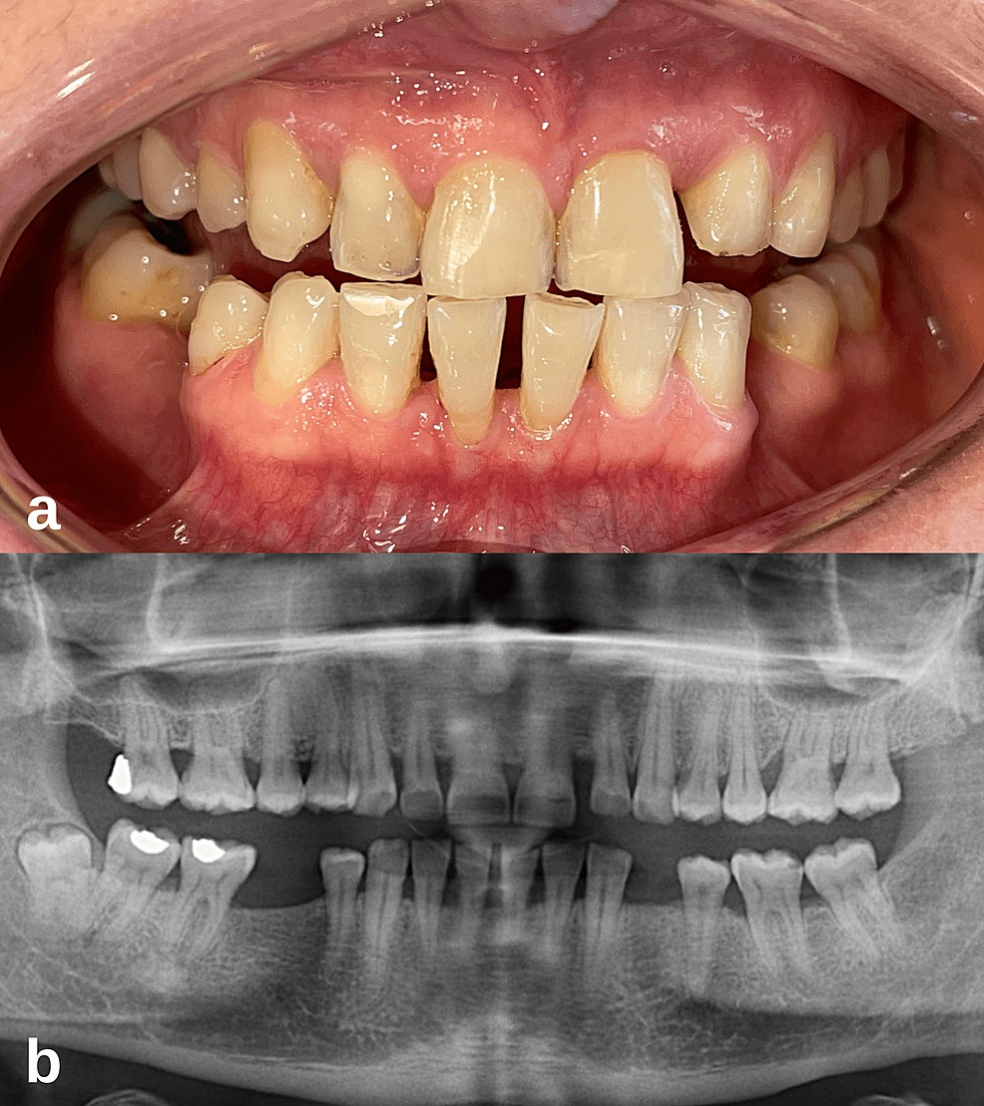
Case 1 initial periodontal examination. (a) Initial clinical intraoral image. (b) Visualization of interdental bone resorption in radiographic examination.
